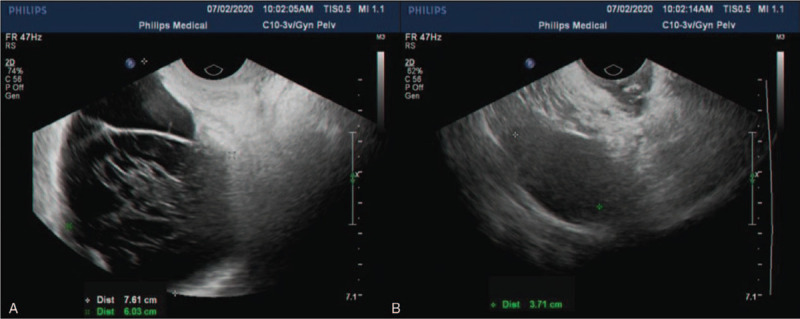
Postoperative gynecological ultrasound of case 1. (A and B) We found a 7.5 cm × 4.9 cm anechoic shape in the left iliac fossa, with an irregular shape, clear boundary, and line-like separation.
